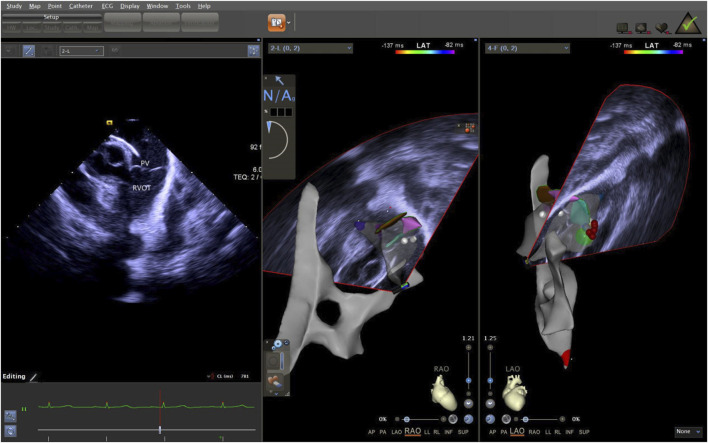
ICE was used to observe the long-axis section of the right ventricular outflow tract. ICE, Intracardiac echocardiography.
